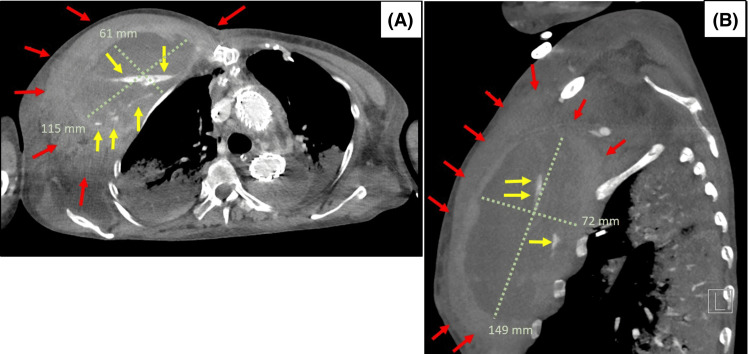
Arterial Phase Chest CT Images: Post-scheduled Surgery (Pre-emergency Surgery) Chest CT images revealed a large hematoma (red arrow) with extravasation (yellow arrow) significantly compressing the right thoracic rib cage. The light green lines indicate the measurements of the chest hematoma. (A) Axial view of CT imaging of chest wall hematoma. (B) Sagittal view of CT imaging of chest wall hematoma.
